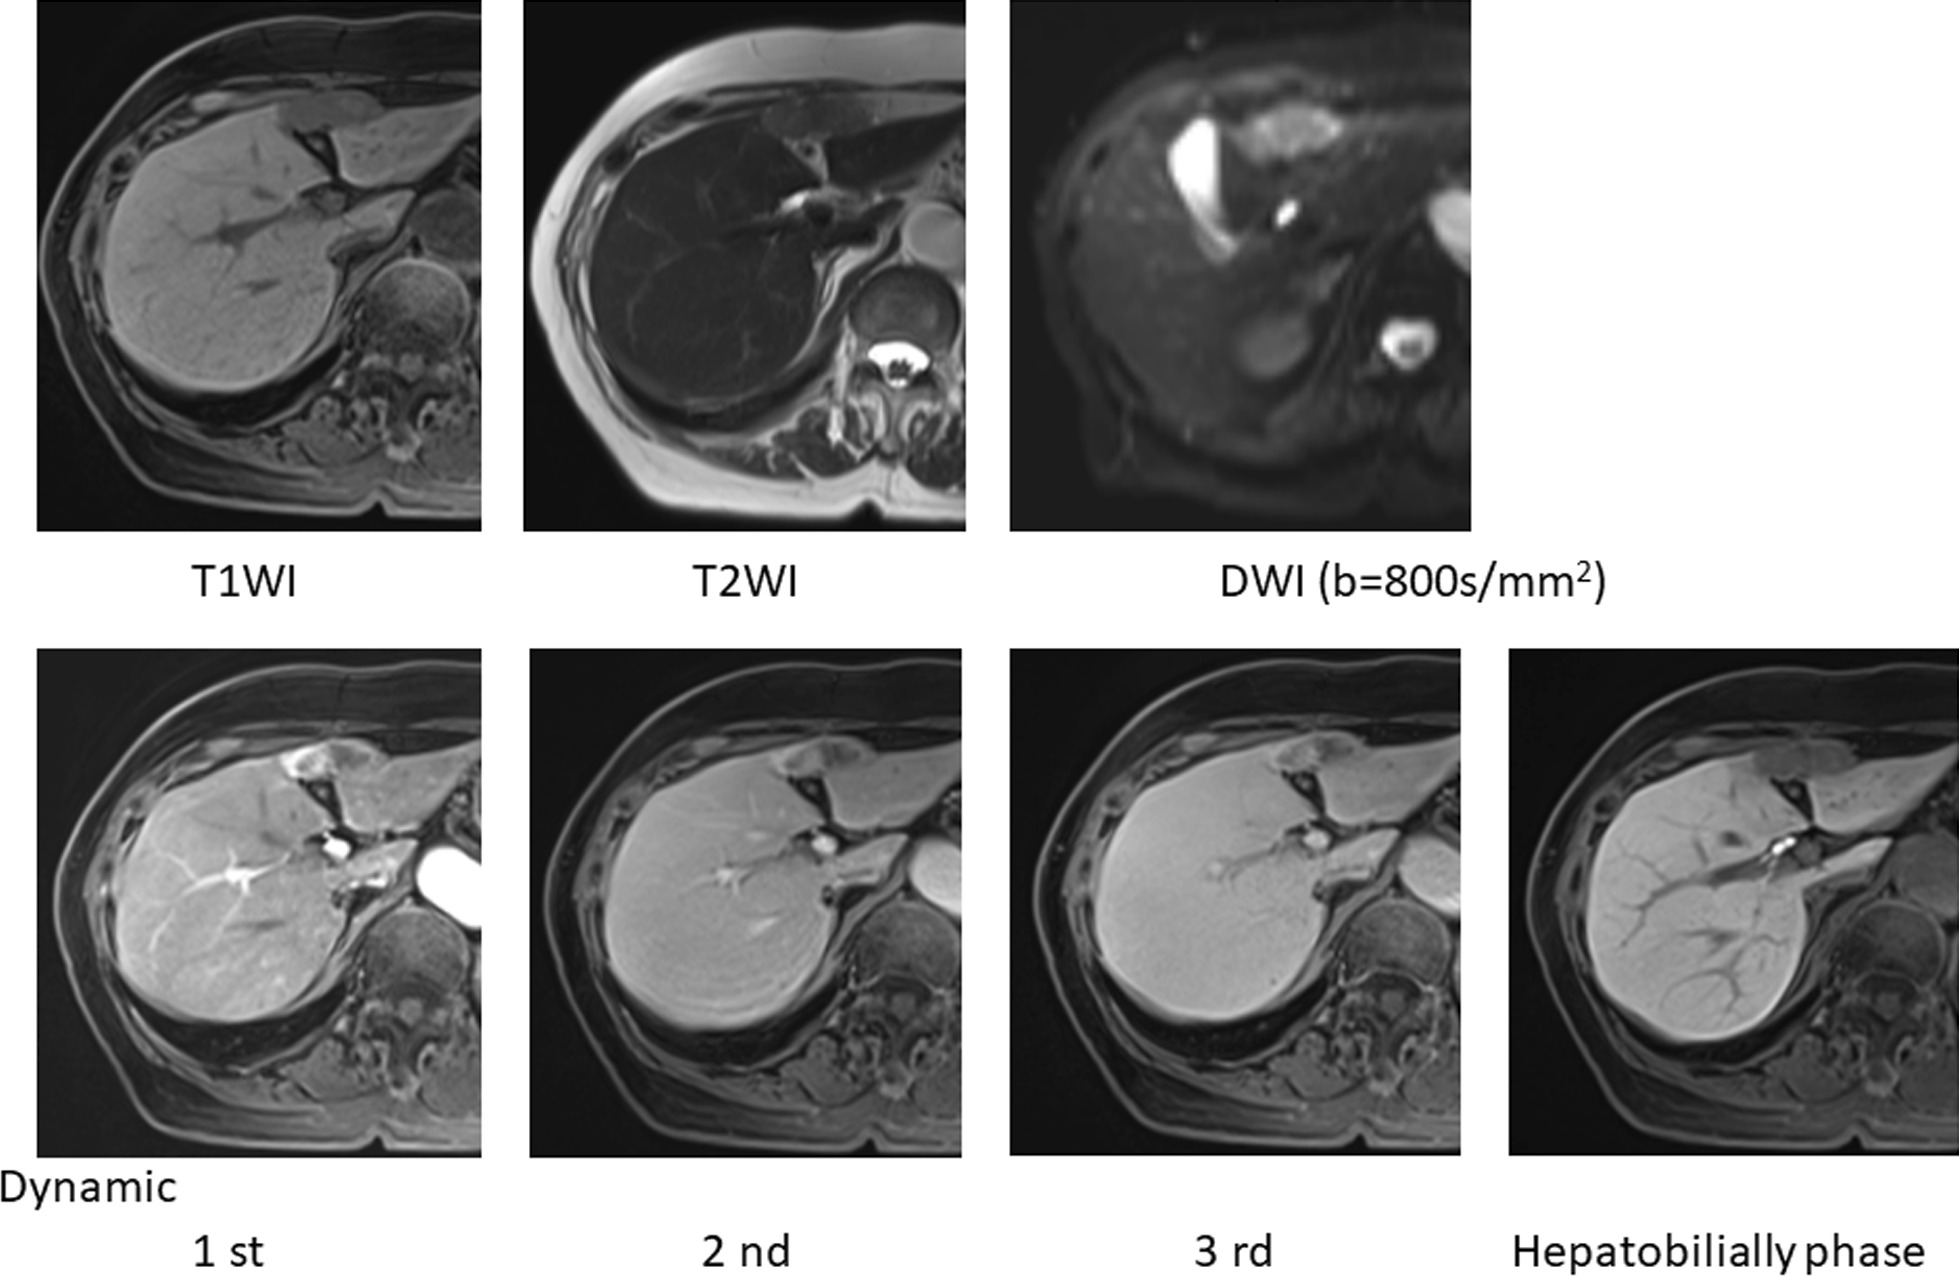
Gadolinium ethoxybenzyl diethylenetriamine pentaacetic acid magnetic resonance imaging images. The images indicate that the tumor has two components. *T1WI* T1-weighted images, *T2WI* T2-weighted images, *DWI* diffusion-weighted images
